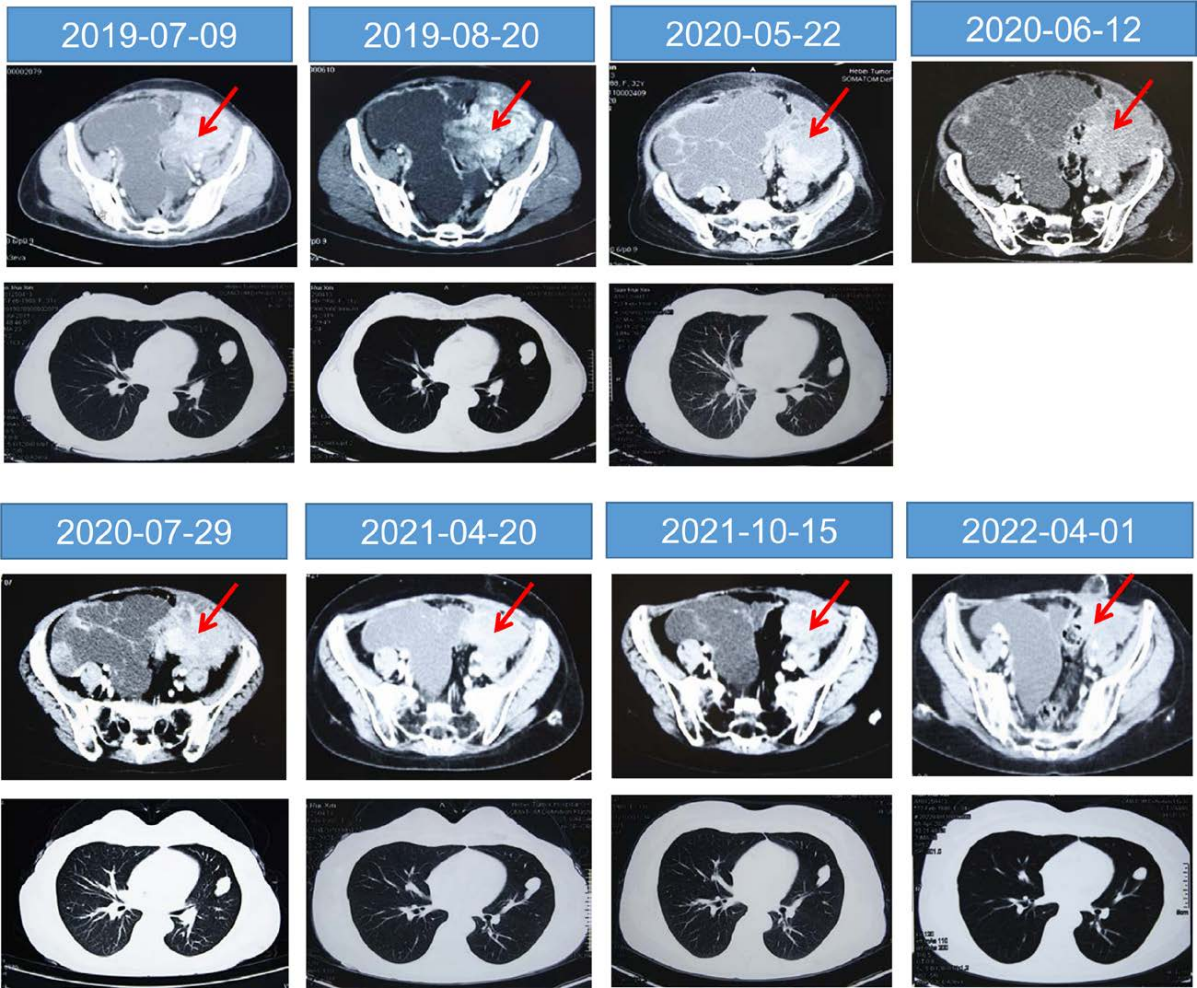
Computed tomography scan showing the changes of recurrent space-occupying lesion (as shown by the arrows) in abdominal cavity (upper panels) and the metastatic tumors in lung (lower panels) during the treatment.
